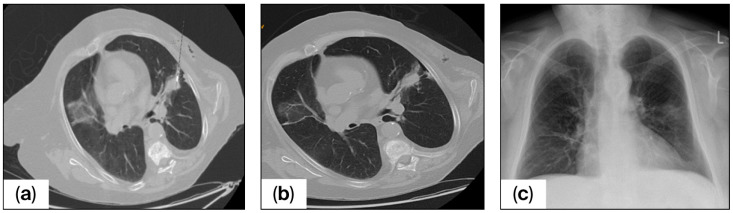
Axial non-contrast chest CT images in the lung window of a patient with a suspected pulmonary nodule in the left upper lobe. (a) The needle trajectory with an insertion angle of 48°. (b) Needle withdrawal after the biopsy tract was filled with saline solution, also revealing a small pneumothorax. (c) Chest X-ray performed two hours later showing no pneumothorax, and the patient remained asymptomatic throughout.
